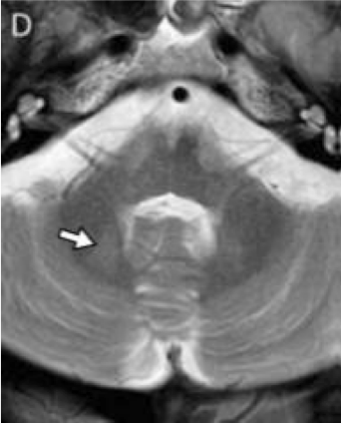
Axial T2-weighted MRI of the brain in a case of hereditary spastic paraplegia 7 (SPG7) showing hyperintensity of the dentate nuclei (arrowed).
